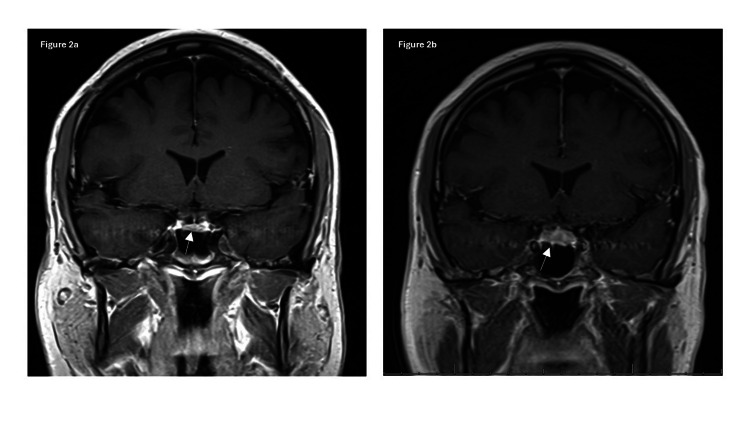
Coronal T1-weighted post-contrast MRI brain images (2a) Showing normal pituitary morphology at baseline (2b) Interval enlargement and enhancement after starting immune checkpoint inhibitor (ICI) therapy during symptomatic presentation of hypophysitis. This view complemented the sagittal imaging and demonstrated thickening of the pituitary stalk and gland expansion.
